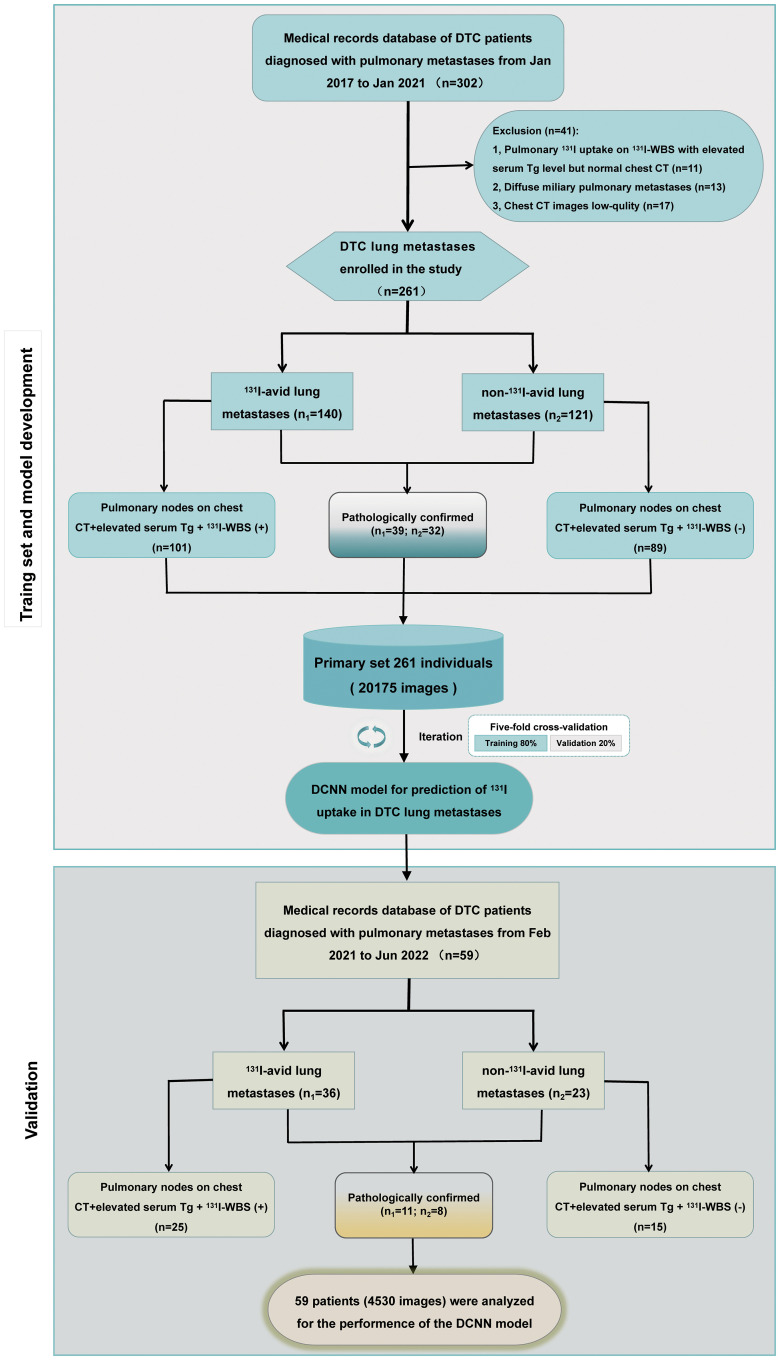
The proposed framework of a parallel multitask RoI head. A whole CT volume is fed into the preprocess module to extract coarse anatomical structures and weights. The input image patch is then sampled via the weights. The backbone and FPN extract multiscale feature maps and feed them into the RPN head. Next, the RPN head proposes RoIs to the parallel multitask RoI head. For each proposed nodule RoI (nRoI), we use a time-scaled nRoI to generate a context RoI (cRoI). The context branch focuses only on the context category classification. For each branch, three parallel subheads perform the same task. Finally, the RoI head classifies whether the proposal is a nodule based on the features of the nodule and context.
